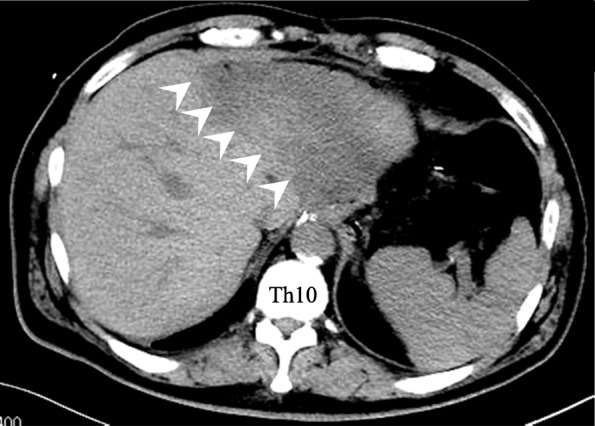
Computed tomography on the fifth postoperative day. CT revealed a low-density area in the lateral segment of the liver indicated by arrowheads, suggesting infarction
